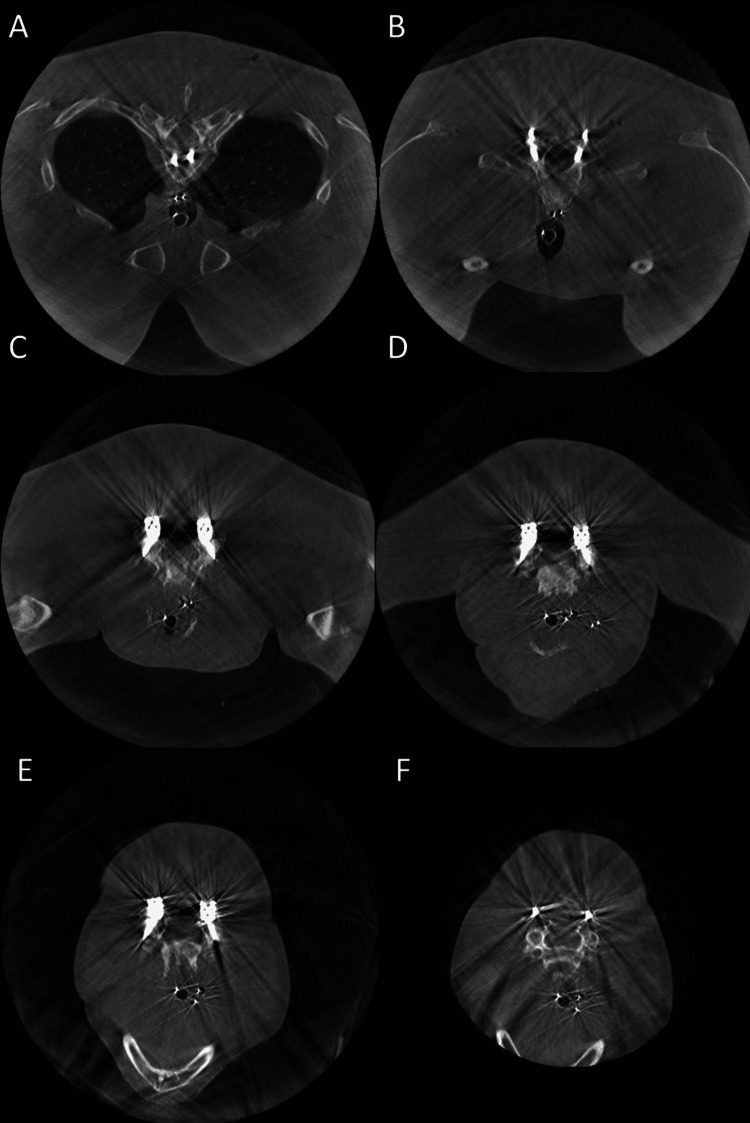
Dyna-CT demonstrating the removal of tethered drain without residual fragments
